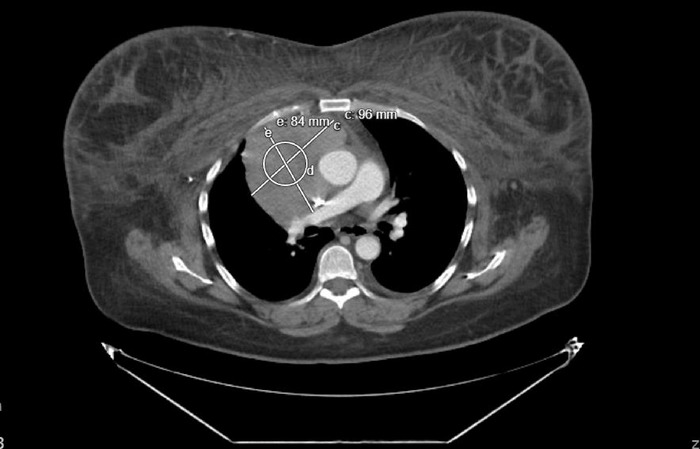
Initial computed tomography scan showing a large anterior mediastinal mass causing superior vena cava compression.
